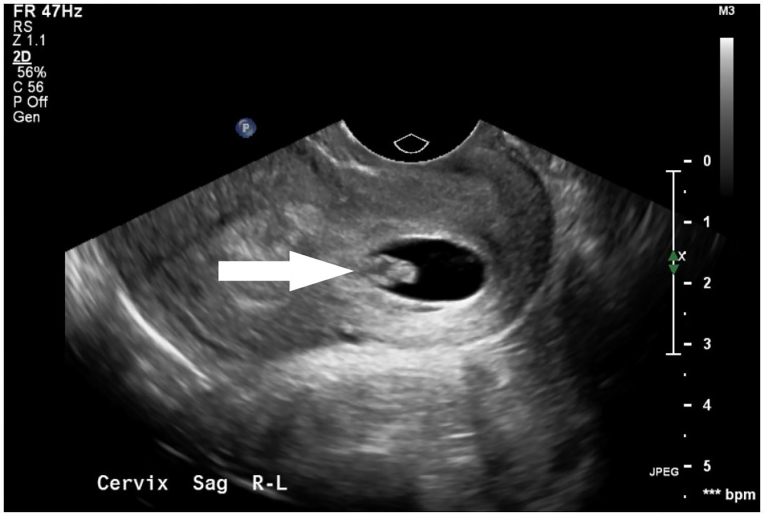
Transvaginal ultrasound demonstrating two uterine cavities (arrows).
